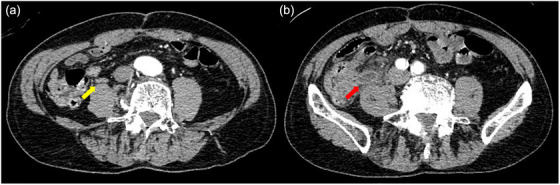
Axial contrast‐enhanced computed tomography images. (a) There is no swelling or thickening of the appendix wall on admission (yellow arrow). (b) Three days after endoscopic band ligation. The appendix exhibited wall swelling and thickening (red arrow)
